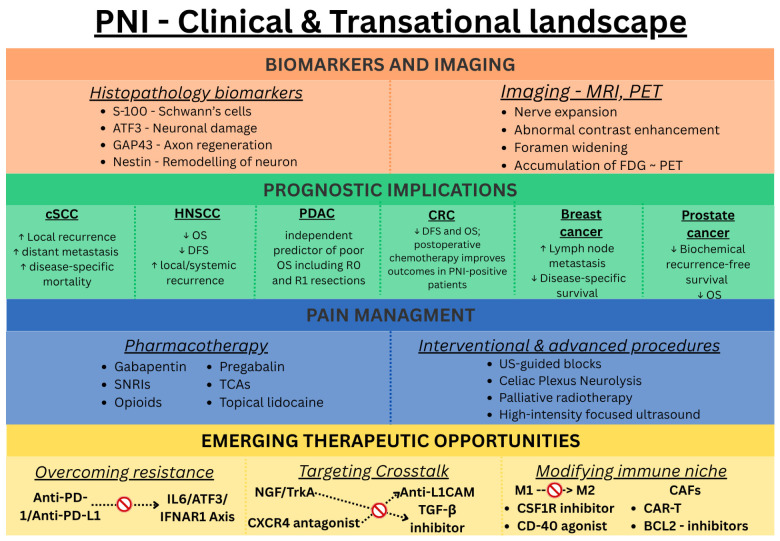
Linking The biology of PNI to clinical practice. Abbreviations: MRI: magnetic resonance imaging; PET: positron emission tomography; ATF3: activating transcription factor 3; FDG: fluorodeoxyglucose; cSCC: cutaneous squamous cell carcinoma; HNSCC: head and neck squamous cell carcinoma; PDAC: pancreatic ductal adenocarcinoma; CRC: colorectal cancer; OS: overall survival; DFS: disease-free survival; SNRIs: serotonin–norepinephrine reuptake inhibitors; TCAs: tricyclic antidepressants; US-guided: ultrasound-guided; PD-L1: programmed death-ligand 1; NGF: nerve growth factor; TrkA: tropomyosin receptor kinase A; CXCR4: C-X-C chemokine receptor type 4; L1CAM: L1 cell adhesion molecule; TGF-β: transforming growth factor β; M1/M2: M1/M2 macrophages; CAFs: cancer-associated fibroblasts; CSF1R: colony-stimulating factor-1 receptor; CAR-T: chimeric antigen receptor T cells.
